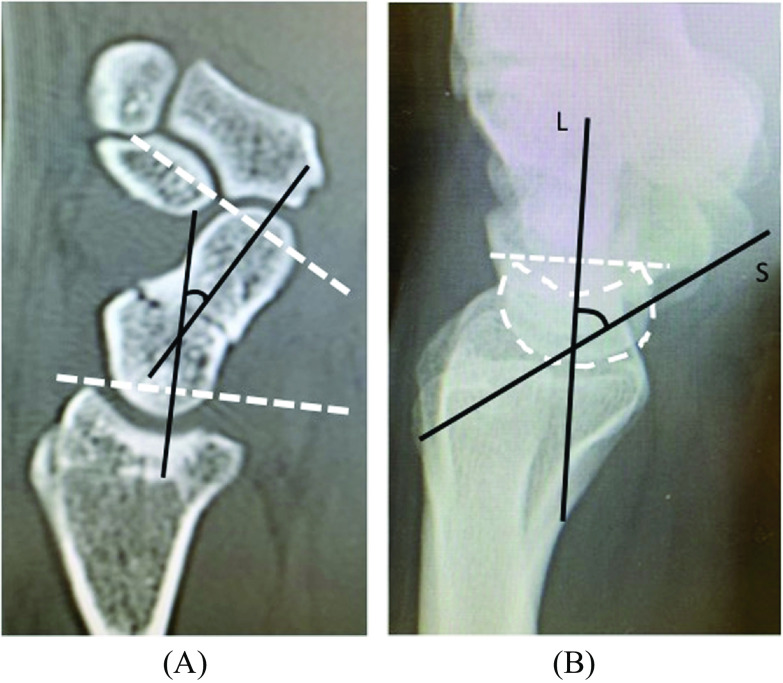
Method for the measurement of the intrascaphoid angle (ISA) (A) and scapholunate angle (SLA) (B). (A) ISA was measured as the angle between the perpendicular lines on the distal and proximal articular surfaces of the scaphoid in the lateral CT image. (B) SLA was measured as the angle between the long axis of the scaphoid (tangent on the volar cortex of the scaphoid) and the long axis of the lunate (axis connecting the midpoints of the proximal and distal arcs) in the lateral view of plain radiography of the wrist.
